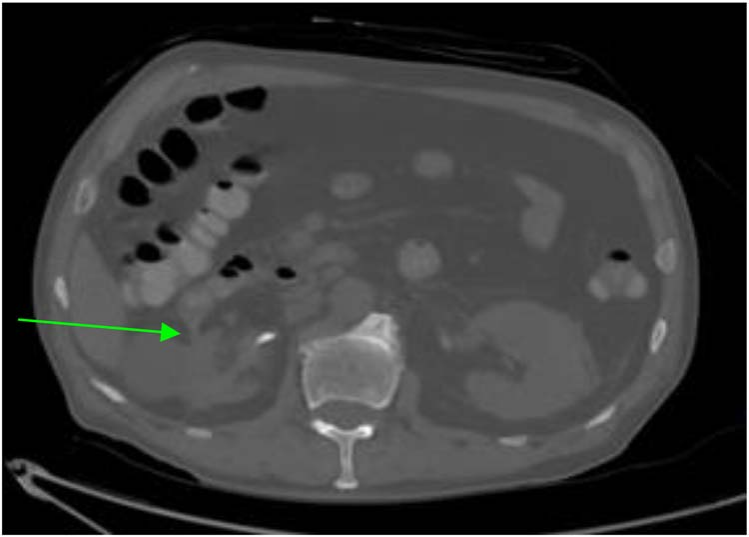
CT abdomen and pelvis revealing a right sided renal-duodenal fistulous connection.
